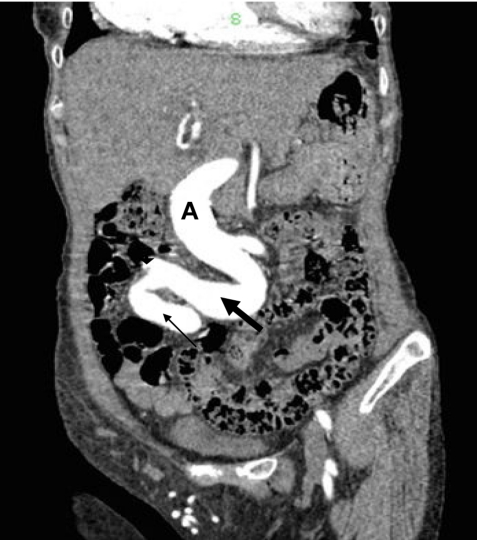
Coronal reconstruction of CT scan shows tortuous atherosclerotic aneurysmal abdominal aorta (A), right common (thick arrow) and internal iliac arteries (thin arrow) and origin of the right external iliac artery (arrowhead).
